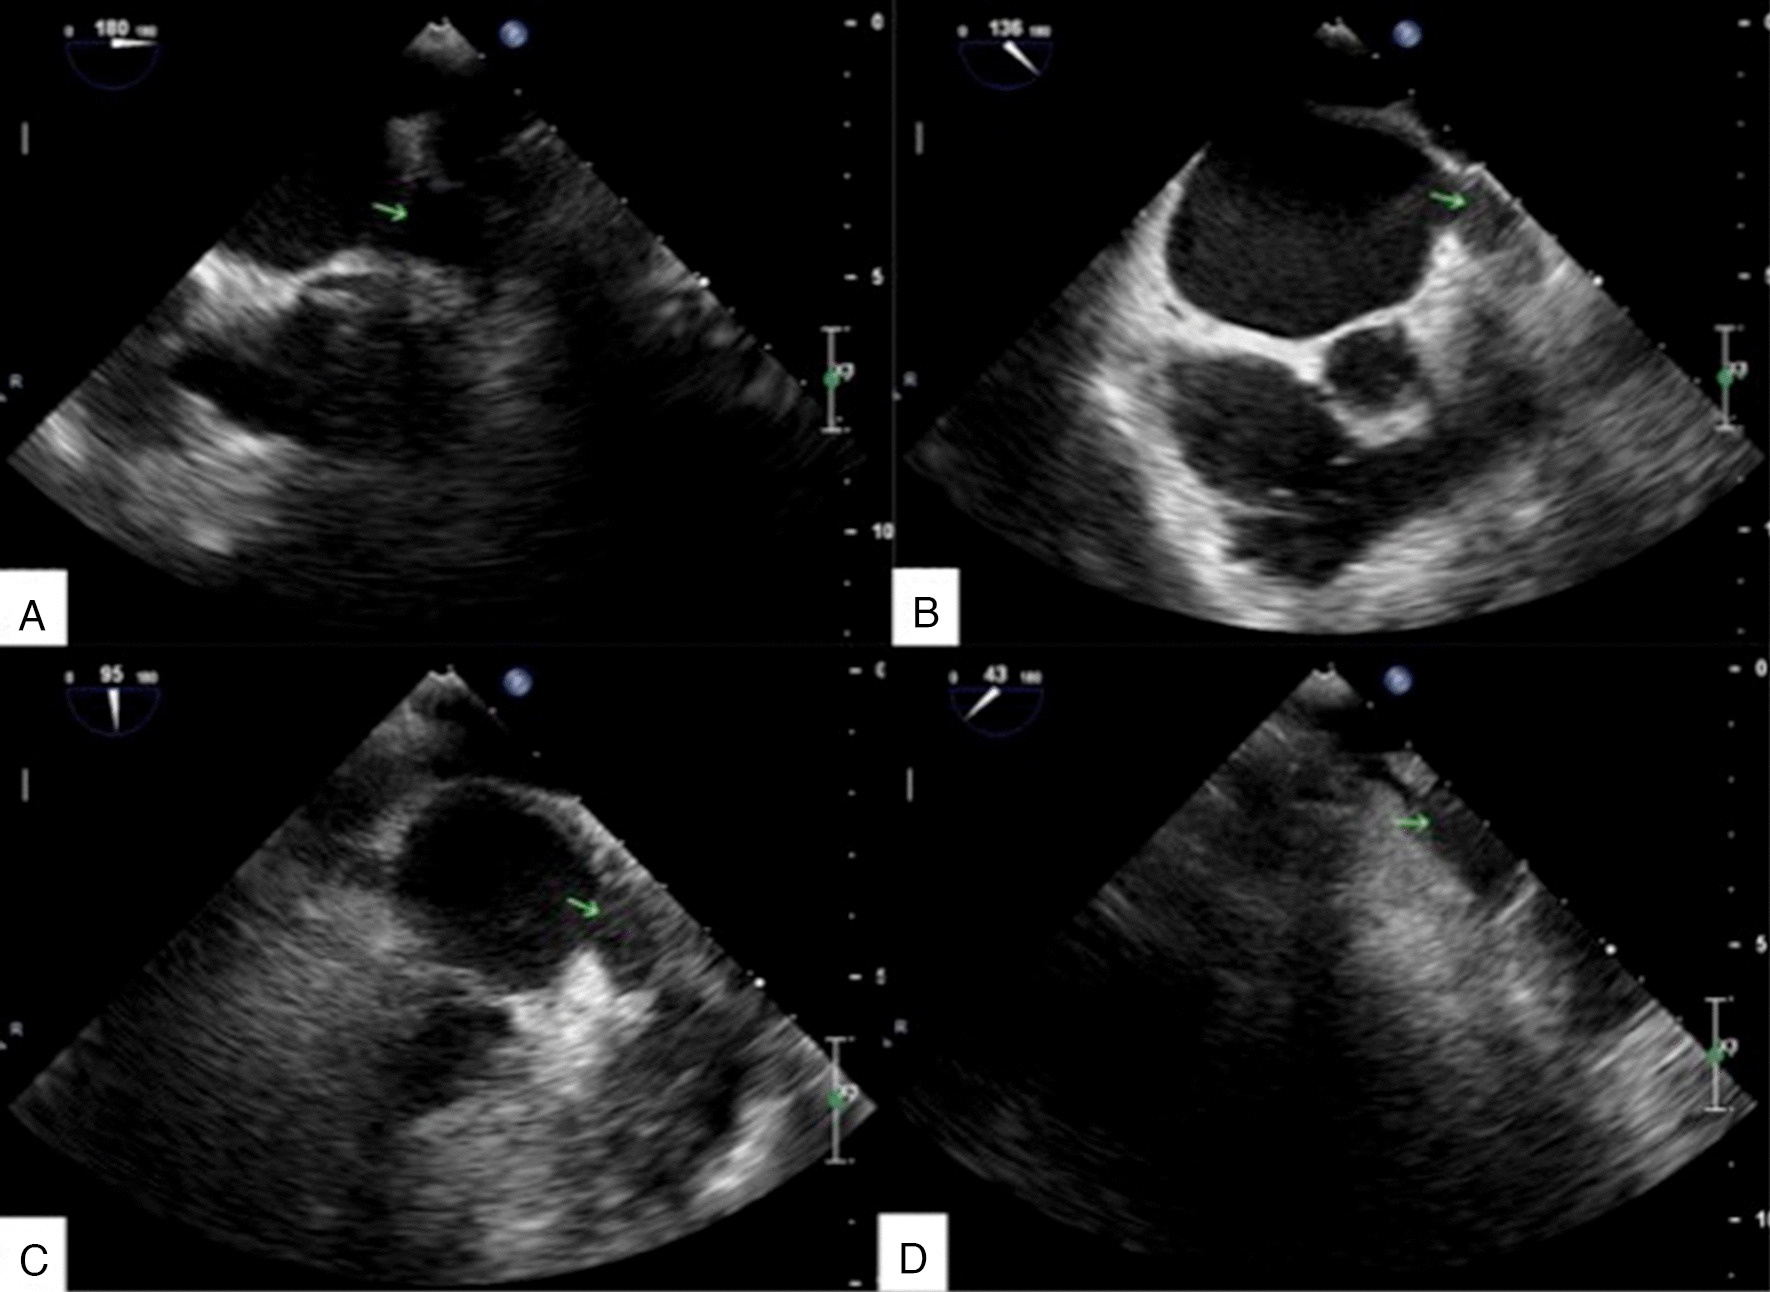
Transesophageal echography (TEE) images in the corrected angles of 0° (A), 45° (B), 90° (C), and 135° (D). The arrows show the left atrial appendage, clean without thrombosis
